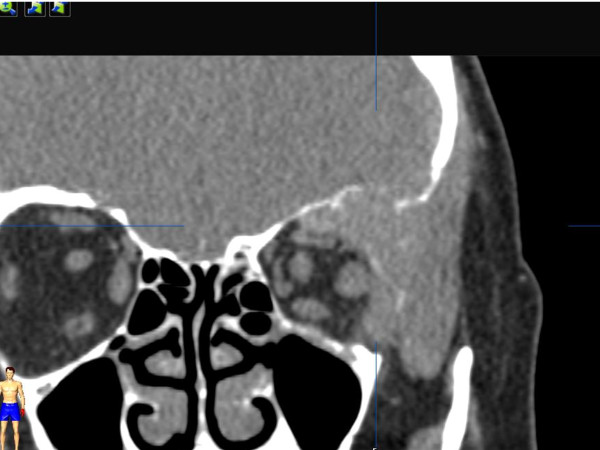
The coronal CT scan reveals large tumor mass of the left orbit with bony destruction of orbital roof and lateral orbital wall as well as skullbase infiltration.
